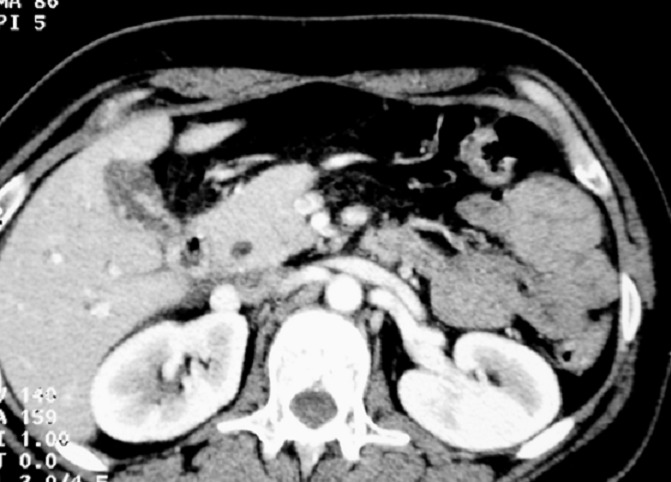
Gallbladder aspect in CT abdominal scan
